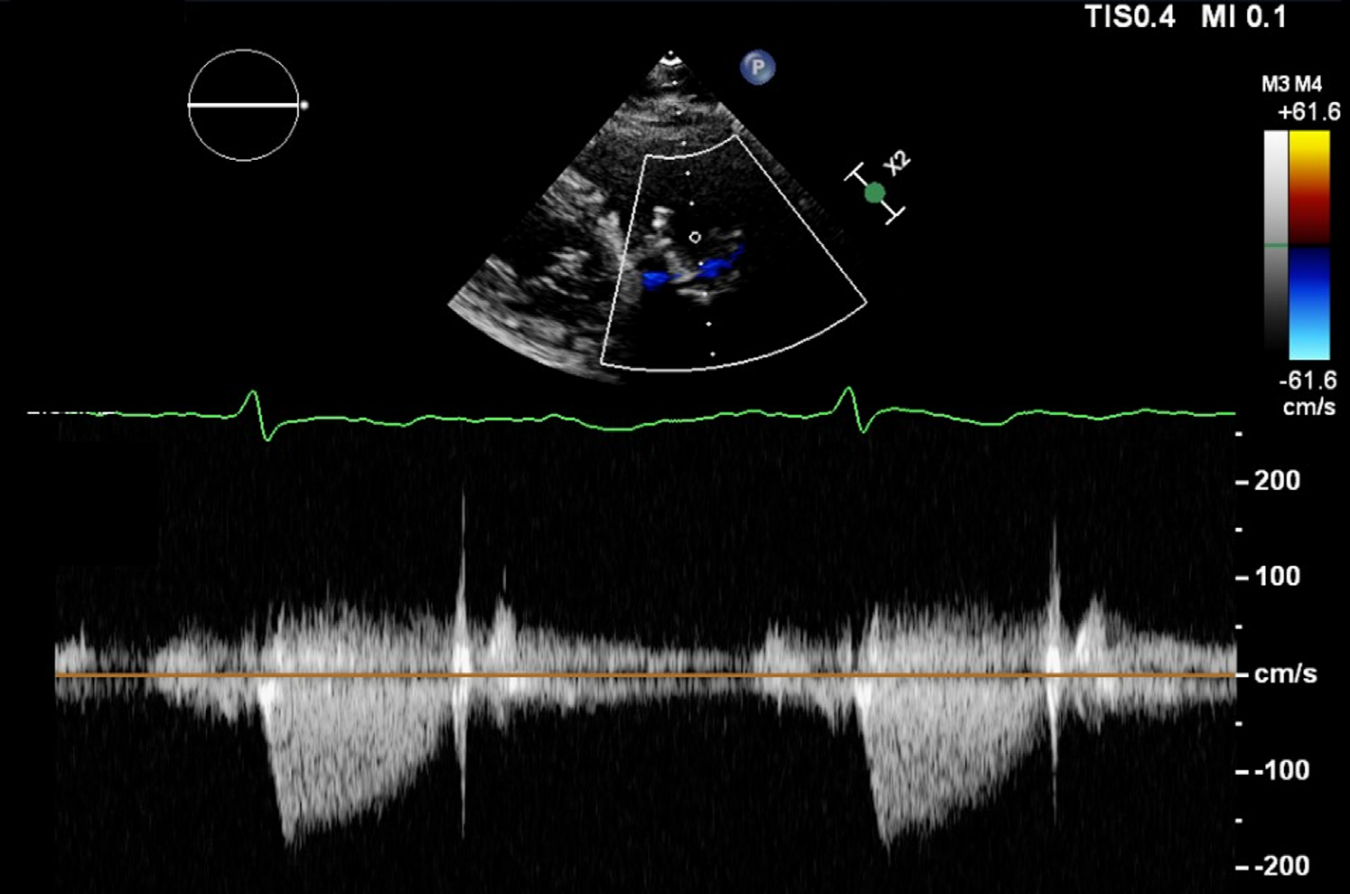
Transthoracic Echocardiogram Continuous Wave Doppler Across the Pulmonary Valve Imaging reveals a mean gradient of 6.1 mm Hg.
